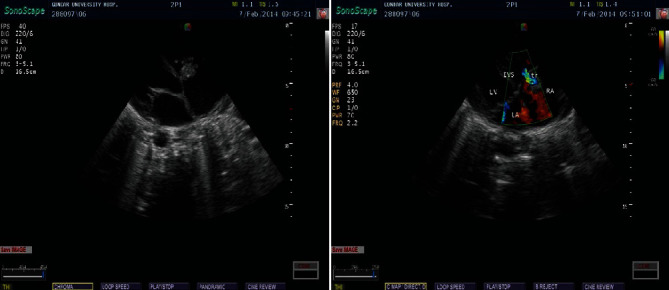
Doppler echocardiography showing an echogenic mobile focus (2.4 cm by 1.7 cm) attached to the anterior cusp of the valve (a) with moderate tricuspid regurgitation (b).
